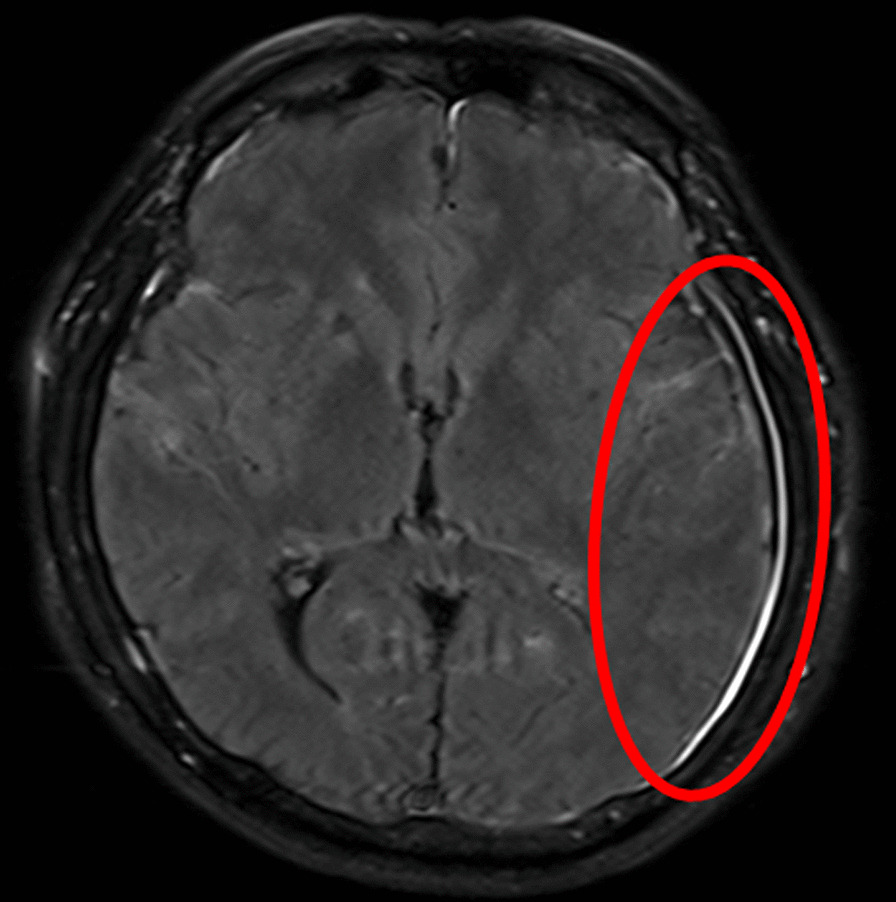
Head contrast-enhanced MRI showing meningeal thickening and contrast enhancement (red circle)
